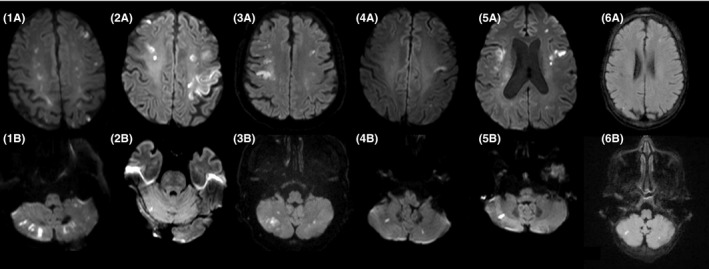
Diffusion‐weighed imaging of stroke secondary to cancer. Two images (A: supratentorial and B: infratentorial) for each of the six cases. Notice DWI lesions in cerebellum and cerebrum
